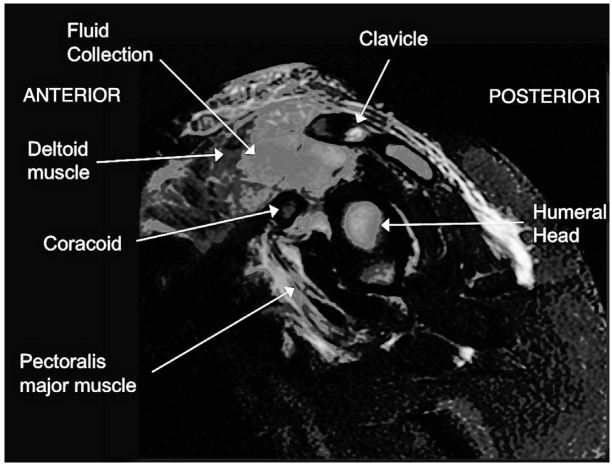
Sagittal magnetic resonance imaging of the left shoulder shows a multiloculated fluid collection within the anterior head of the deltoid and distal pectoralis major muscles.
